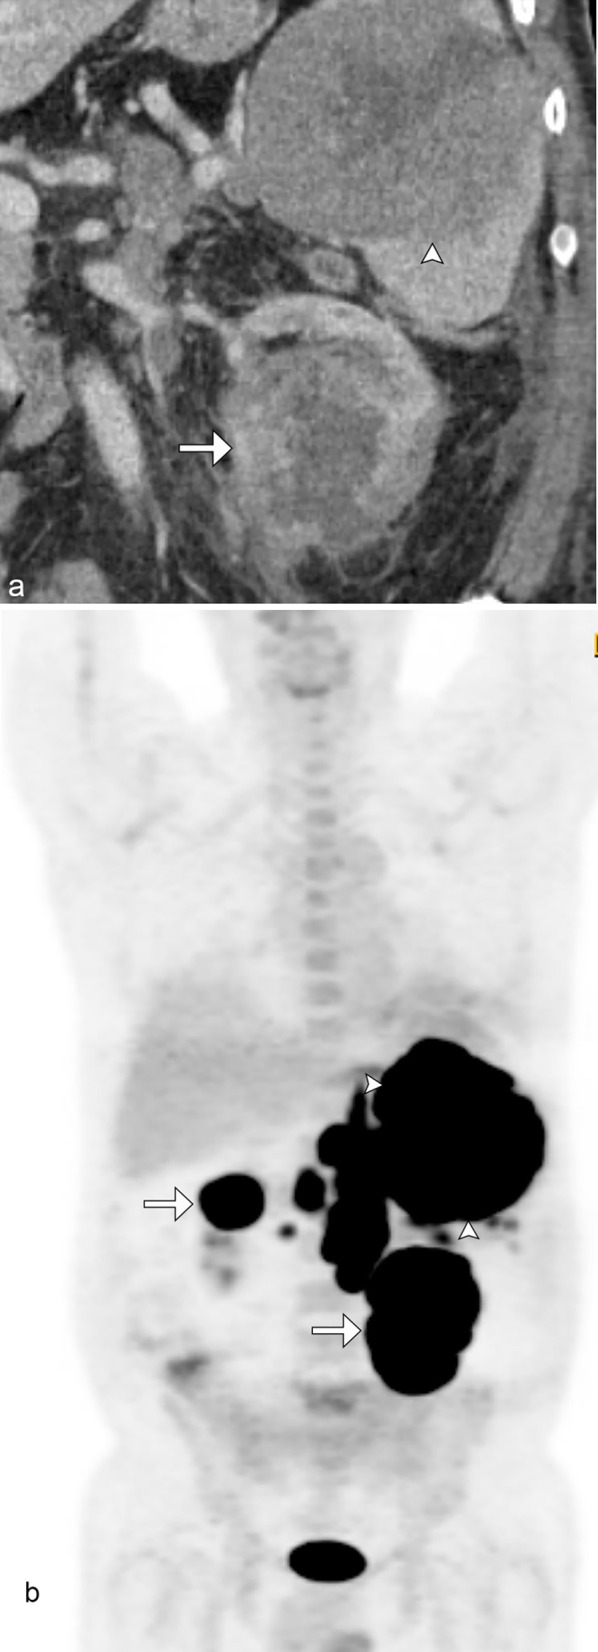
Solitary mass of renal lymphoma.* Coronal CT post contrast image (a) demonstrates a solitary hypoenhancing left lower pole renal mass (arrow). Note the mass-like infiltration of the spleen (arrowhead) and retroperitoneal lymphadenopathy. PET/CT (b) shows the marked F18-FDG avid bilateral renal masses (arrows), retroperitoneal lymphadenopathy, and splenic mass (arrowheads), consistent with the diagnosis of large b-cell lymphoma
